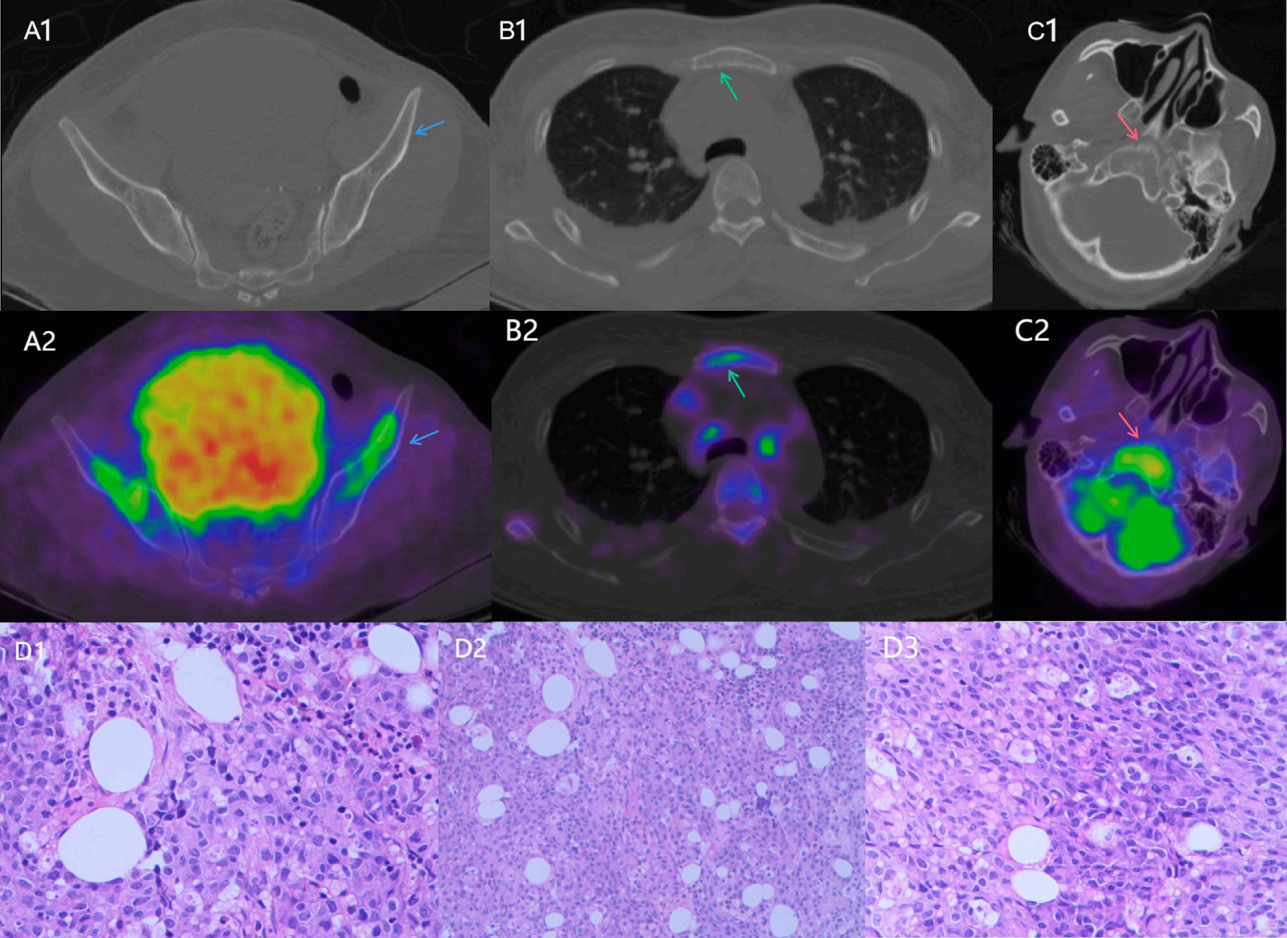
^18^F-FDG PET/CT images of the patient with BM from GC showed extensive osteolytic changes of bone lesions. The axial images A1, B1, and C1 are CT only; A2, B2, and C2 are fused PET/CT. Bone destruction with abnormal FDG uptake (SUVmax = 3.7) was detected in the left ilium (A1, A2, blue arrows), sternum (B1, B2, green arrows) and clival osseous (C1, C2, red arrows). Pathological results of the biopsy of bone lesions showed flaky or focally distributed tumor cells in the bone marrow tissue (D1, D2, D3).
